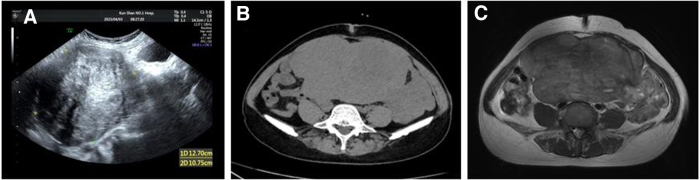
IVL radiographic image. (A) Transvaginal ultrasound was performed 3 days before surgery. (B) Axial CT scan of the abdomen showing a large pelvic mass. (C) MRI images showing a large mass of uterine origin that was initially considered a uterine sarcoma. CT, computed tomography; IVL, intravascular leiomyoma; MRI, magnetic resonance imaging.
